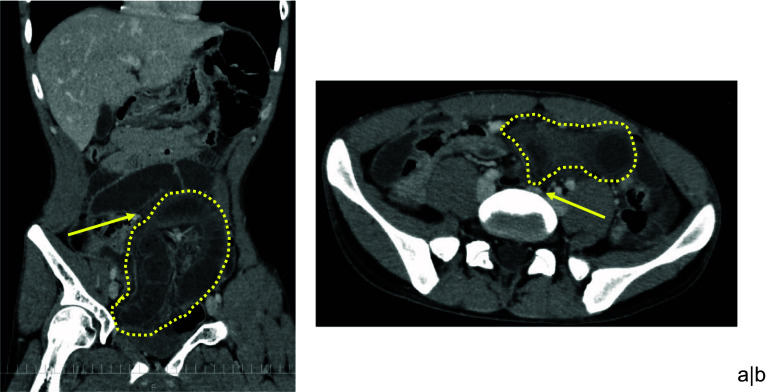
CT scan findings. a A closed loop (dotted line) formed in the left lower abdomen. The obstruction point was at the midline of the abdomen (arrow). b Contrast-enhanced imaging showed intestinal edema with a partially non-enhanced area. A closed loop (dotted line) and the site of obstruction (arrow) were identified
